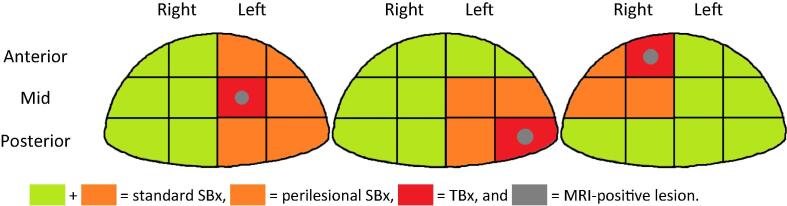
Graphical representation of TBx, TBx + standard SBx, and TBx + perilesional SBx. MRI = magnetic resonance imaging; SBx systematic biopsies; TBx = targeted biopsies.
